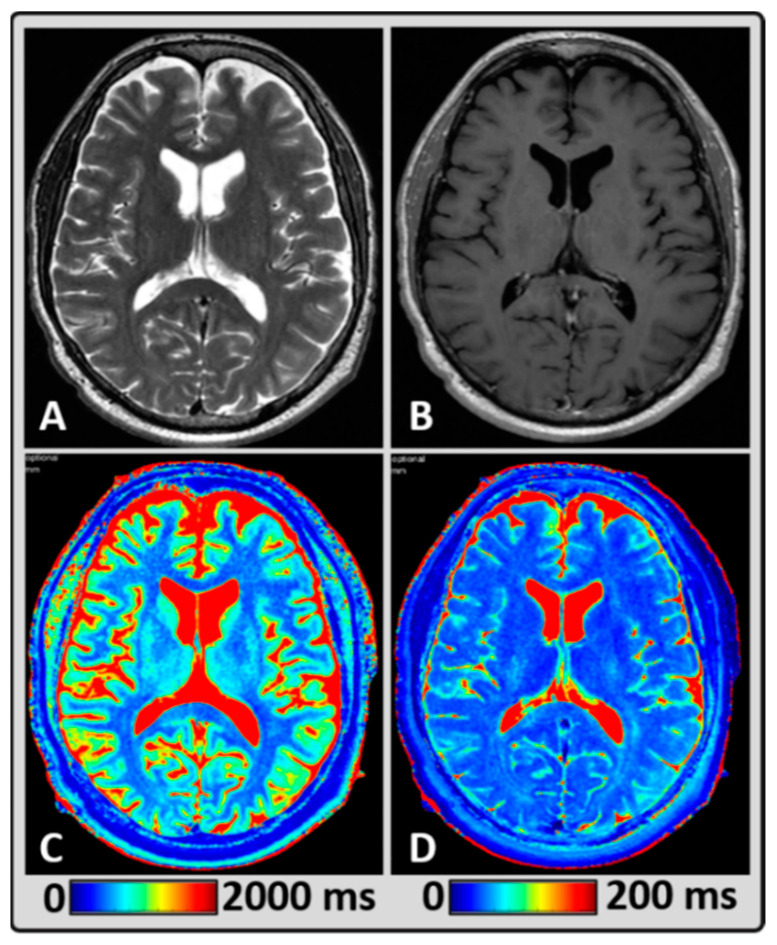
Representative MRI data from a 58-year-old male BM patient treated with focal radiation therapy (RT), showing normal-appearing brain tissue. (A,B) standard T2w and post-contrast T1w images depicting the anatomical structures, (C,D) T1 and T2 maps were estimated using MAGnetic resonance image Compilation (MAGiC) for a single slice exhibiting gray matter (GM), white matter (WM), and cerebrospinal fluid (CSF).
